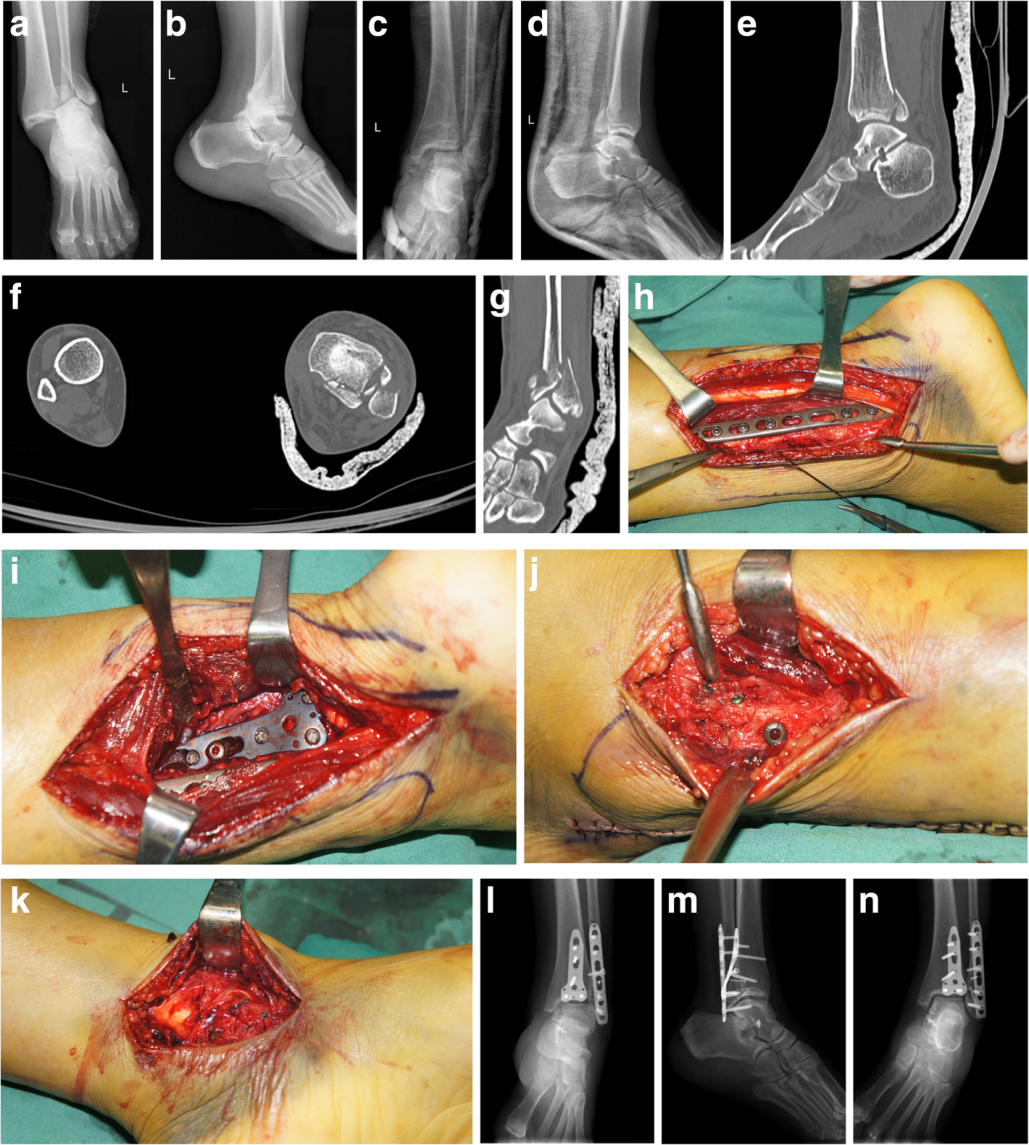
Pre-reduction (a anteroposterior; b lateral) and postreduction (c anteroposterior; d lateral) radiographs from a 52-year-old woman after falling down (untypical Logsplitter injury). The CT scans (e, f, g) indicated peroneal Wagstaffe-Le Fort fracture and posterior Volkmann fracture. During the surgery, fibular (h) and Volkmann fragments (i) were fixed using the plates; Wagstaffe fragments (j) were fixed using the cannulated screws; triangular ligament and medial soft tissues were restored (k). The anteroposterior (l), lateral (m), and mortise (n) radiographs at the final follow up showed good reduction
